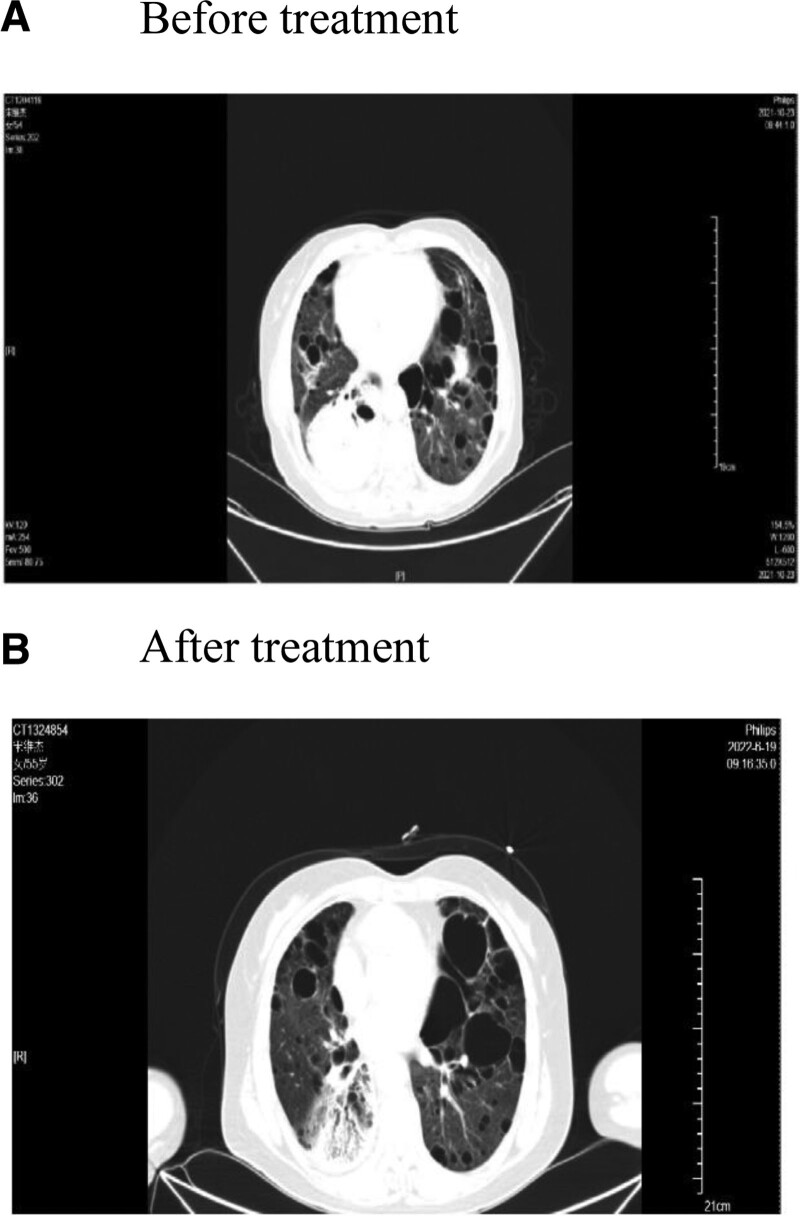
Computed tomography scan shows that the soft tissue density mass in the lower lobe of the right lung was flaky and had a slight high-density shadow. (A) Before treatment and (B) after treatment.
